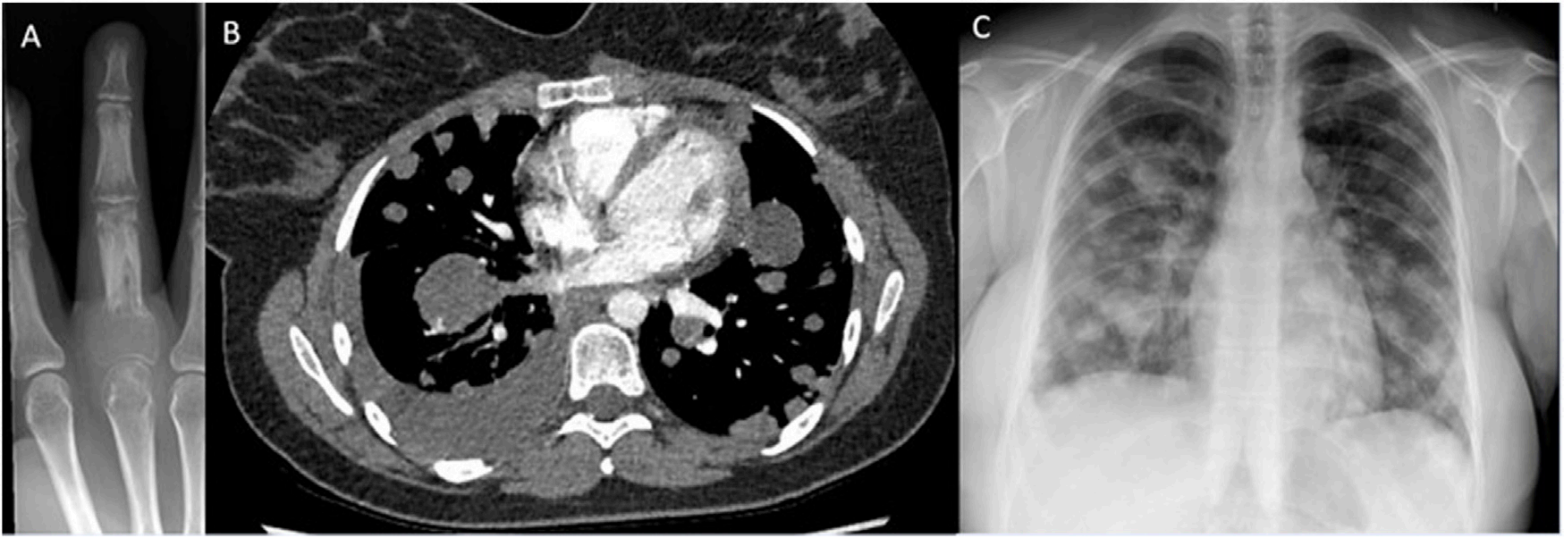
Imaging of GCTB from one of the patients. (A) – X-ray right middle finger 24/09/2018, of primary GCTB tumour. (B) – CT chest, soft tissue window 13/05/2020, showing pulmonary metastatic spread, (C) – X-ray chest 24/08/2020, showing metastatic spread.
